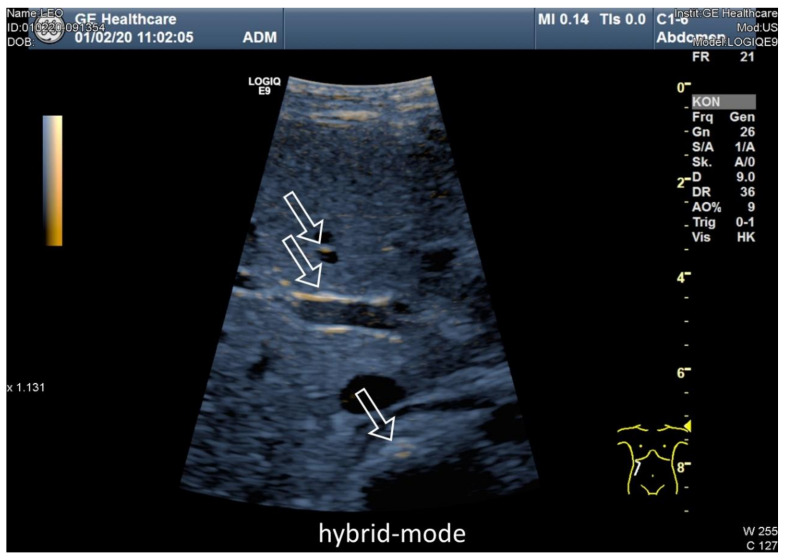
Ultrasound image of liver. Visualization of the microbubbles with the hybrid subtraction mode. Clear enhancement of the bubbles in the larger and smaller liver vessels (arrow).
